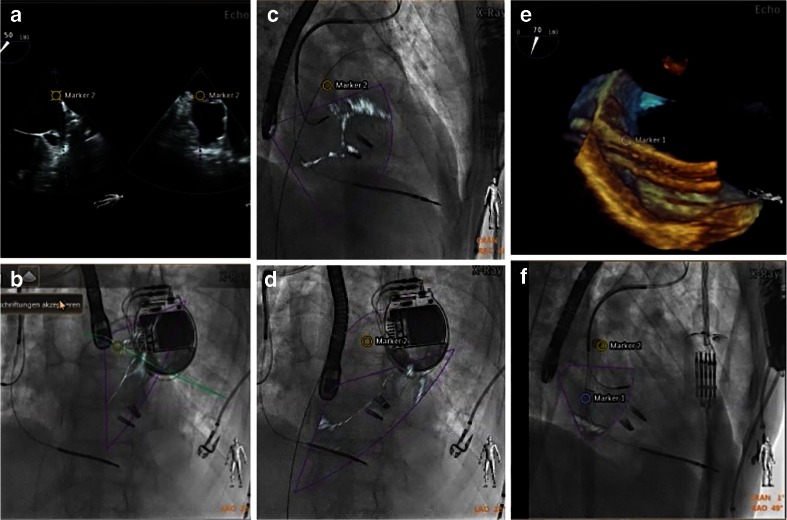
Fusion imaging may improve safety during SHD procedures. On the TEE image, a landmark (*marker 2*) is placed to identify the warfarin ridge on fluoroscopy (a, b). During the procedure, this critical landmark may not always be visible on echocardiography. Nevertheless, it is shown on the fluoroscopy image, with the position updated depending on the C-arm angulation (c LAO 44°, CRA 9°; d LAO 46°, CRA 12°). Such markers ensure safe and precise passage of a large catheter through the interatrial septum (e, *marker 1*) and may raise caution when approaching them (f, *marker 2*)
